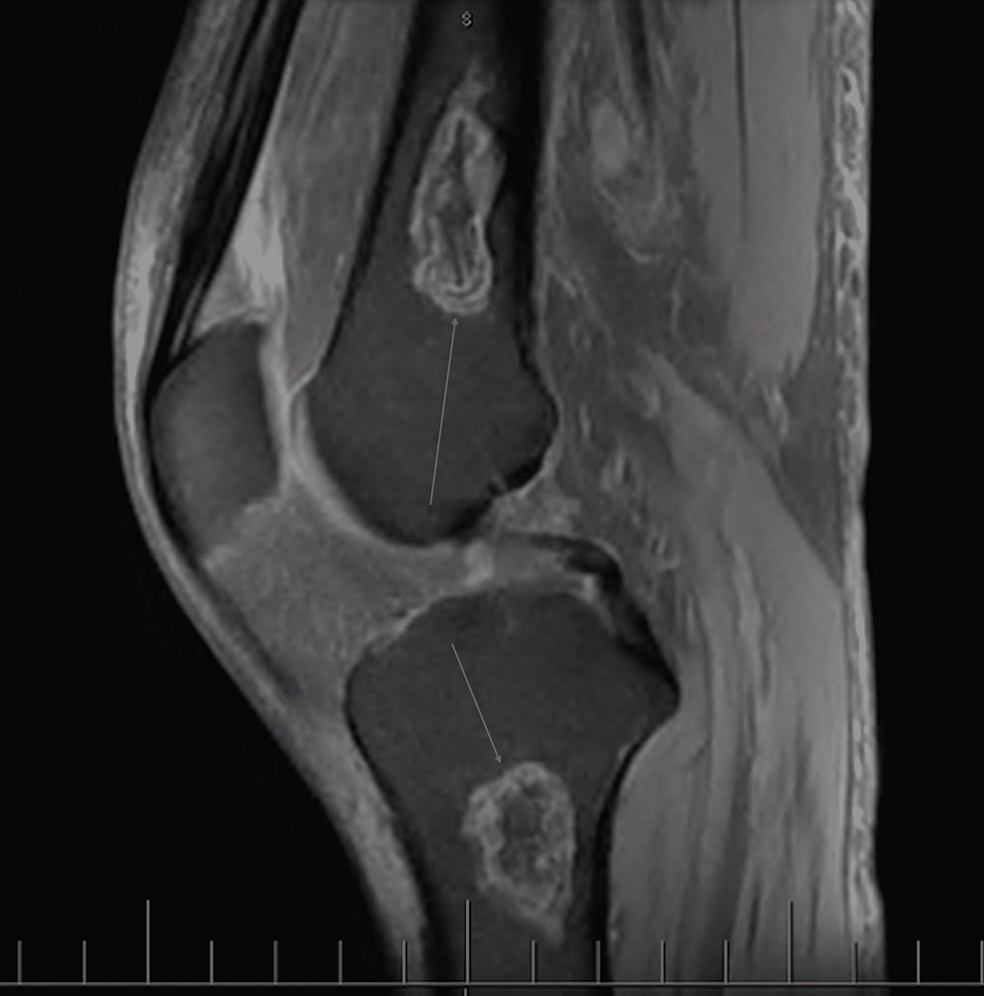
MRI of the left knee with and without contrast revealing bony involvement
